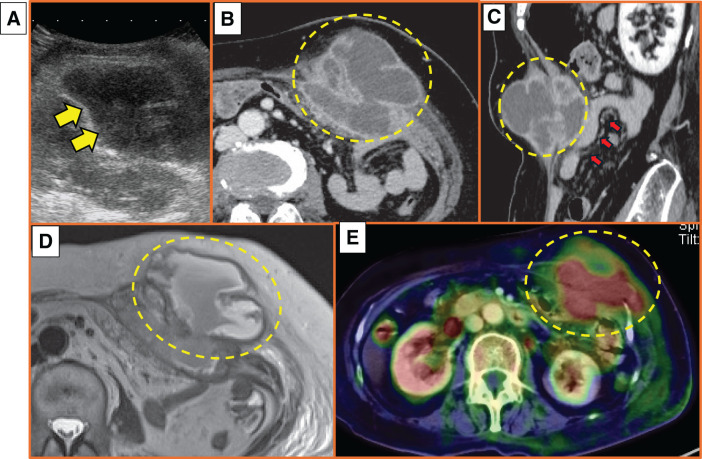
Imaging examinations at the initial visit. (A) Ultrasound shows a heterogeneous, mosaic-like internal structure (yellow arrows). (B) Enhanced CT (horizontal) shows an 8-cm low-density tumor in the left side of the abdominal wall (yellow dotted circle). (C) Enhanced CT (sagittal) shows that the tumor is in contact with the small intestine (yellow dotted circle; red arrows indicate the small intestine). (D) Dynamic MRI shows that the tumor has high signal intensity on T2-weighted imaging (yellow dotted circle). (E) Positron emission tomography-CT shows fluorodeoxyglucose uptake corresponding to the solid component of the tumor. The mass was suspected to have spread into the abdominal cavity, a finding suggestive of malignancy (yellow dotted circle).
